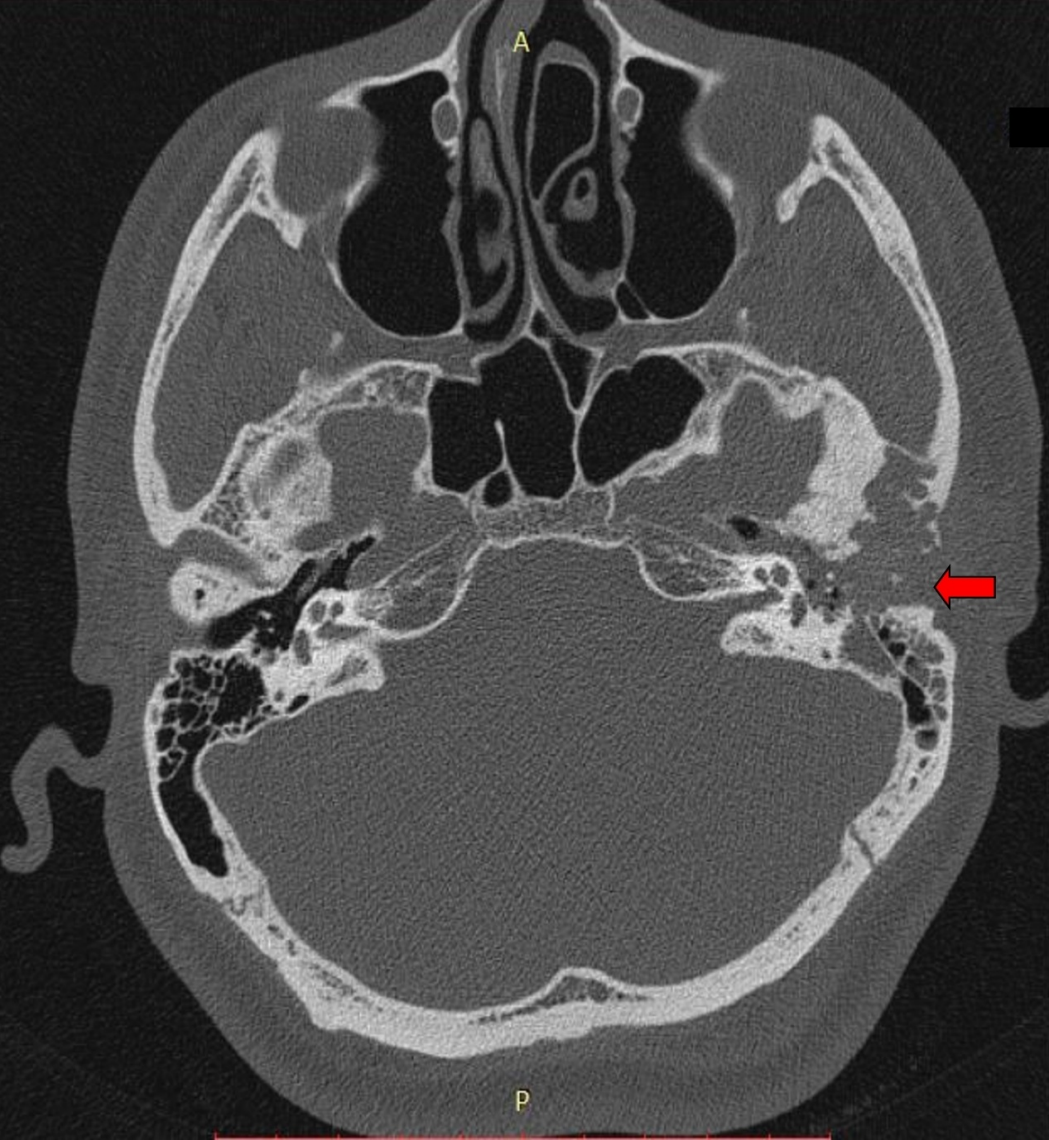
High-resolution computed tomography (HRCT) of temporal bone in axial view showing lesion occupying left external auditory canal and middle ear cleft, and eroding adjacent areas.
